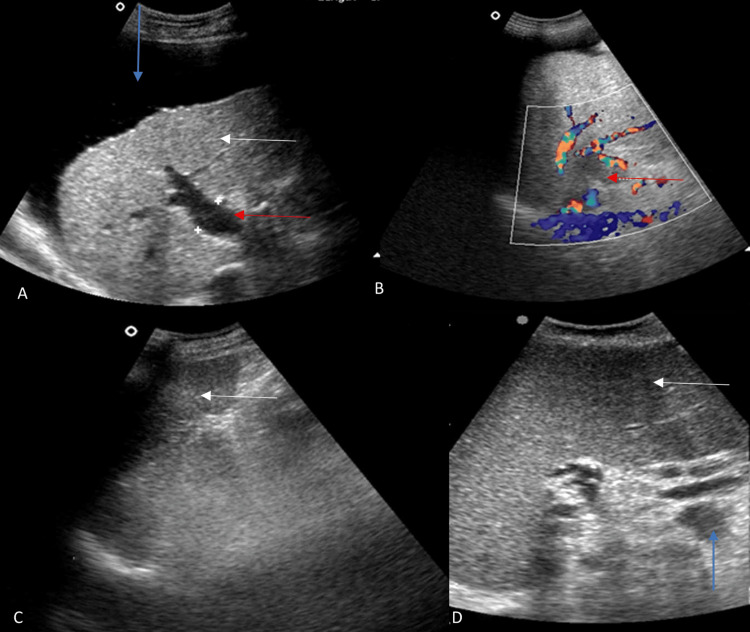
(A) Grey scale image of the liver shows small size, coarse echotexture and surface nodularity of the liver suggesting cirrhosis of liver (white arrow), dilated portal vein (red arrow) and ascites (blue arrow). (B) Colour Doppler image of the portal vein shows no colour flow in the portal vein suggesting portal vein thrombosis (red arrow). (C and D) Grey scale images of the spleen show enlarged spleen suggesting splenomegaly (white arrows) and multiple splenic hilar collaterals (blue arrow). The images included in this review manuscript are from our own clinical cases and are used with appropriate permissions
